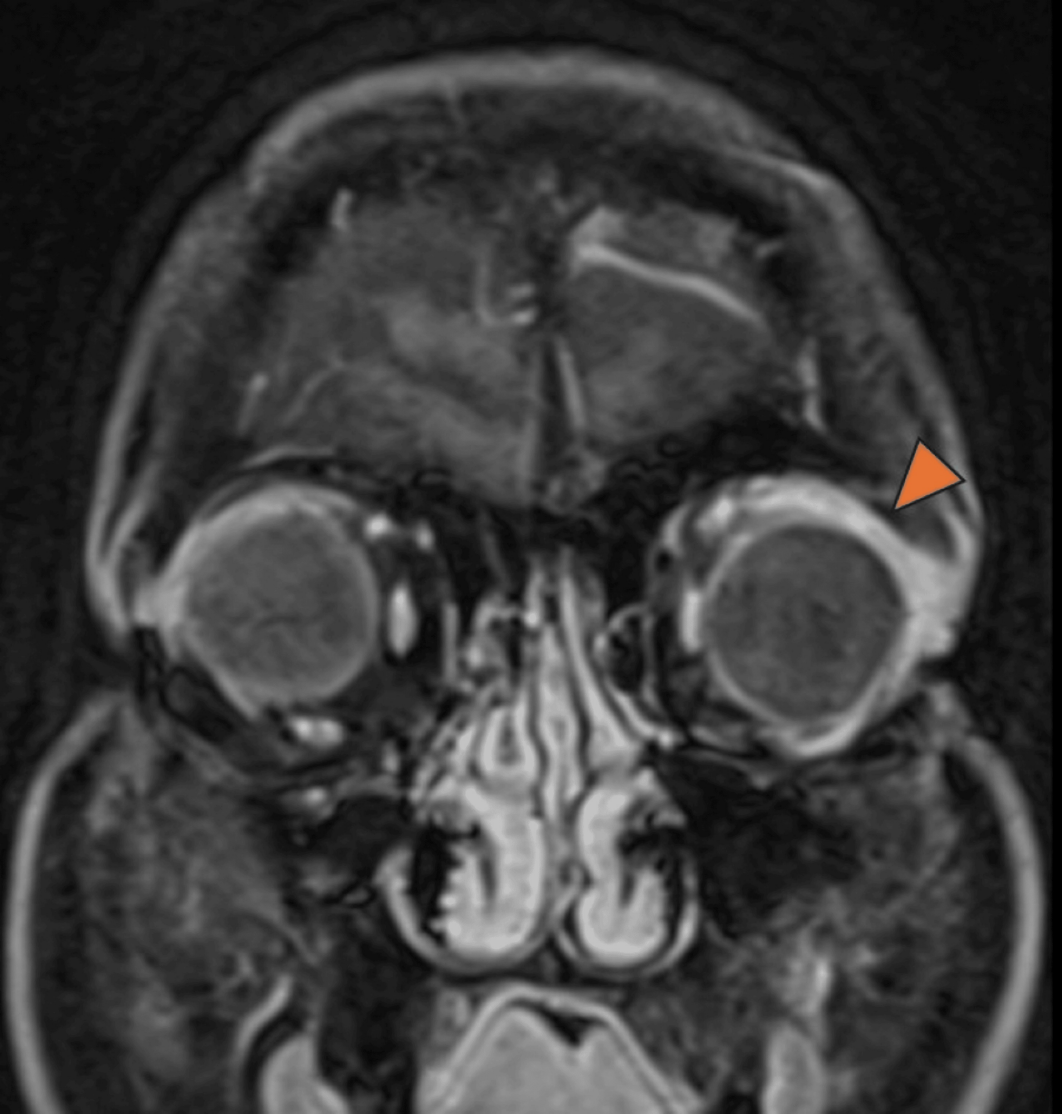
Orbital MRI (coronal T1-weighted sequence) Inflammation of the left lacrimal gland is observed, evidenced by increased signal intensity (hyperintensity) as indicated by the orange arrow. MRI, magnetic resonance imaging
